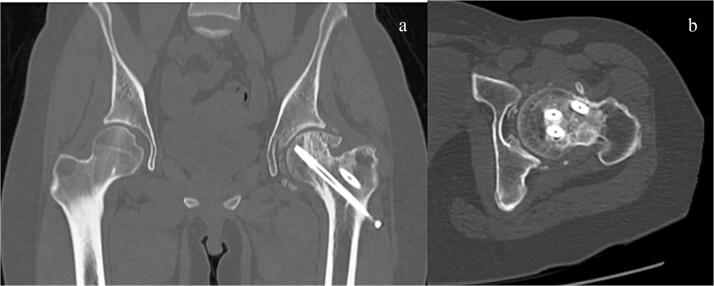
CT imaging revealed a remodeling of the graft bone.
